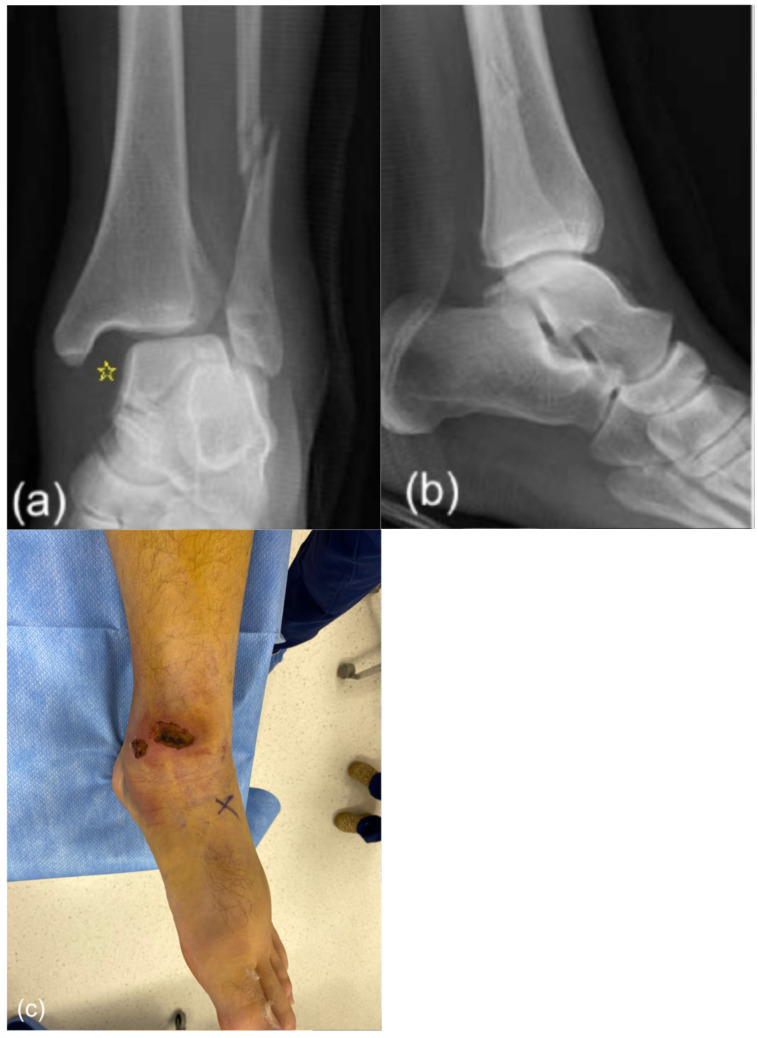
Preoperative AP (a) and lateral (b) radiographs on day 8 showing loss of tibio-talar alignment with widening of medial clear space (asterisk) and syndesmosis; (c) clinical picture showing full-thickness necrosis on the medial side.
